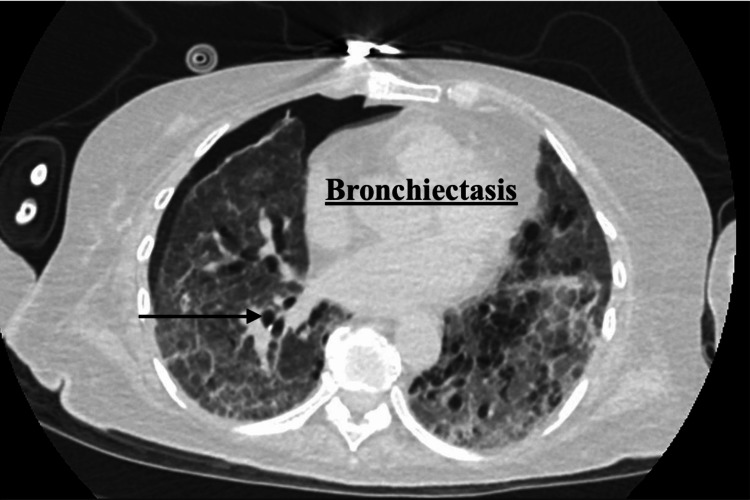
CT scan imaging: bronchiectasis Bronchiectasis is a chronic, irreversible dilation and destruction of the bronchial walls resulting from recurrent infection, inflammation, or impaired mucociliary clearance. For our patient, we hypothesize this injury to be related to radiation therapy received for her breast cancer. CT, computed tomography
